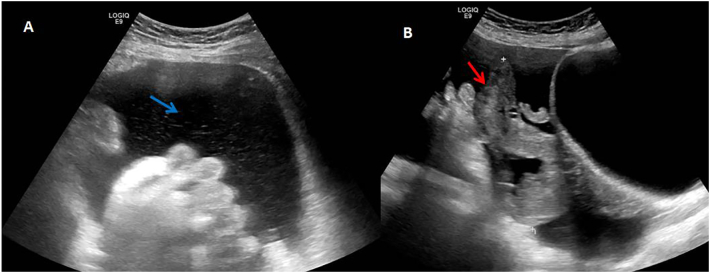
Pelvic ultrasound scans demonstrating moderate volume ascites (panel A, left hand side, blue arrow) and a significant 11 cm in maximal length adnexal mass.
